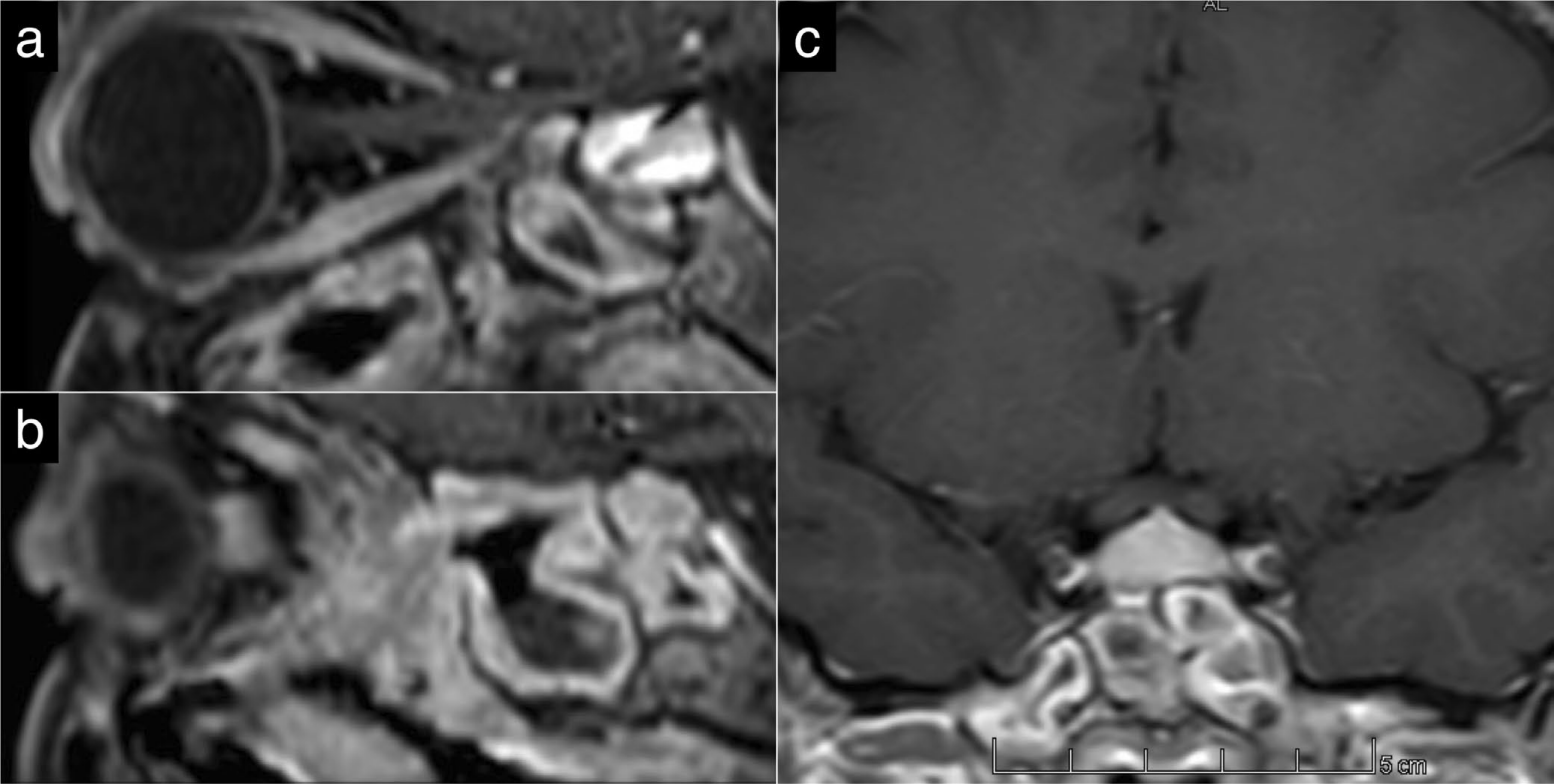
Brain MRI. (a) Enhancement of the pituitary stalk in favor of hypophysitis (sagittal plane); (b) Mucosal thickening of sphenoid sinus in favor of sinusitis; (c) Enhancement of the pituitary stalk and mucosal thickening of the sphenoid sinus (coronal plane).
